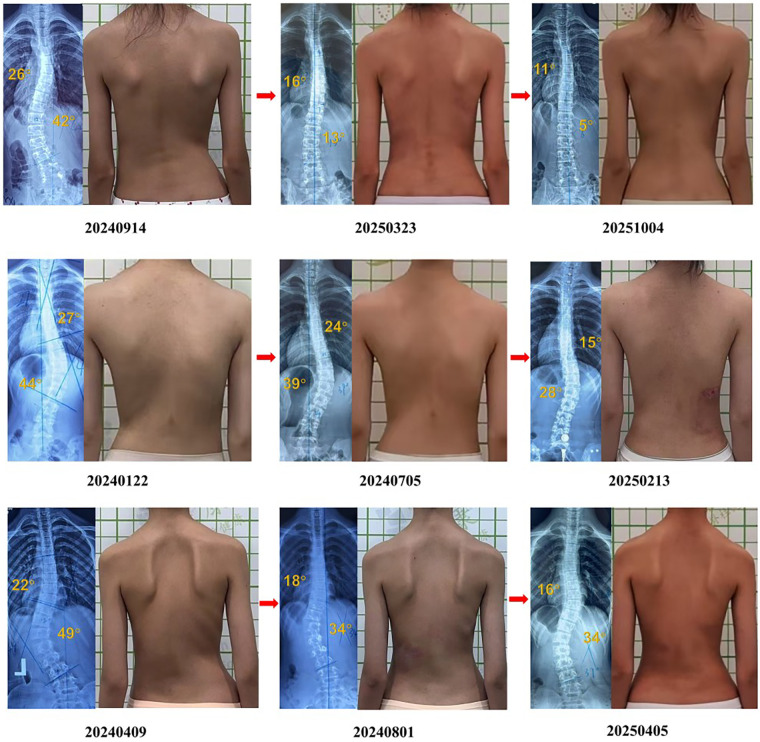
Representative cases demonstrating radiographic cobb angle and clinical surface deformity changes at baseline and after chêneau bracing in patients with severe AIS who declined surgery.
